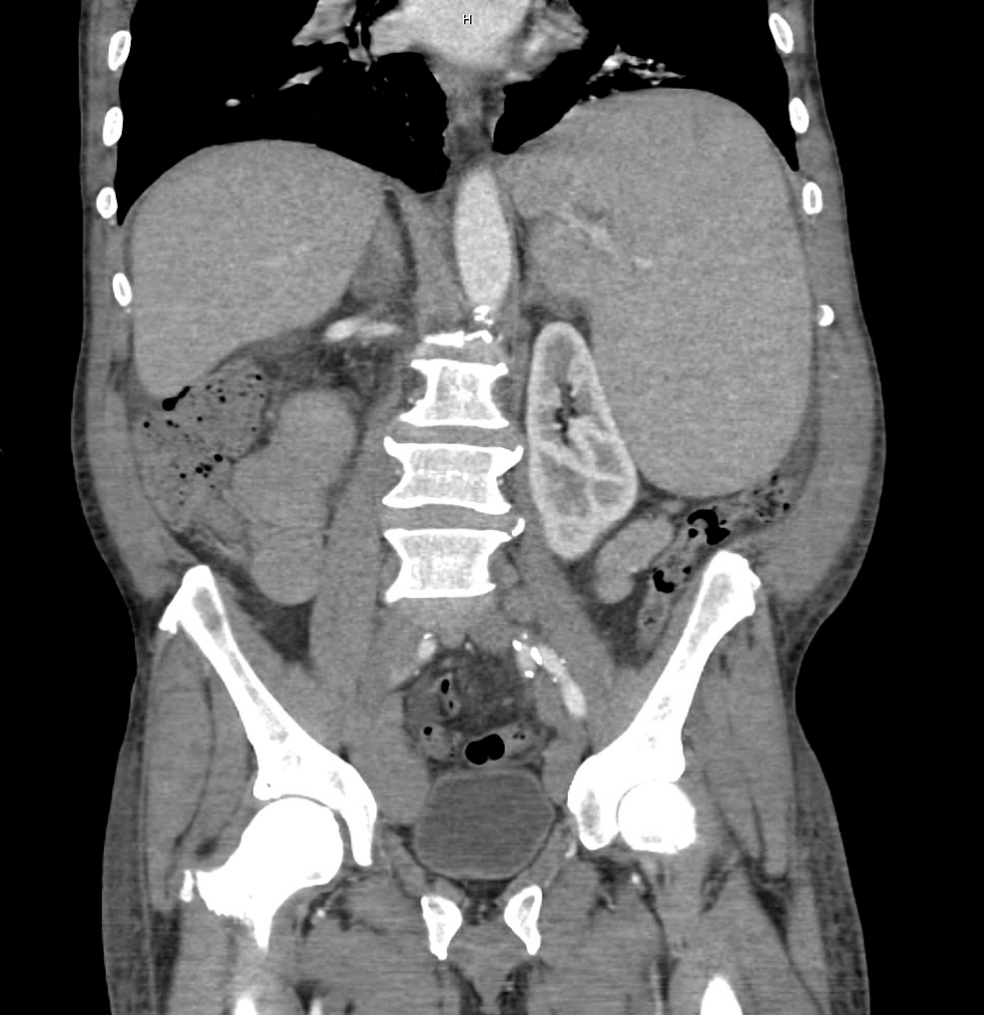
The CT scan of abdomen and pelvis shows bulky lymphadenopathy throughout with massive splenomegaly
